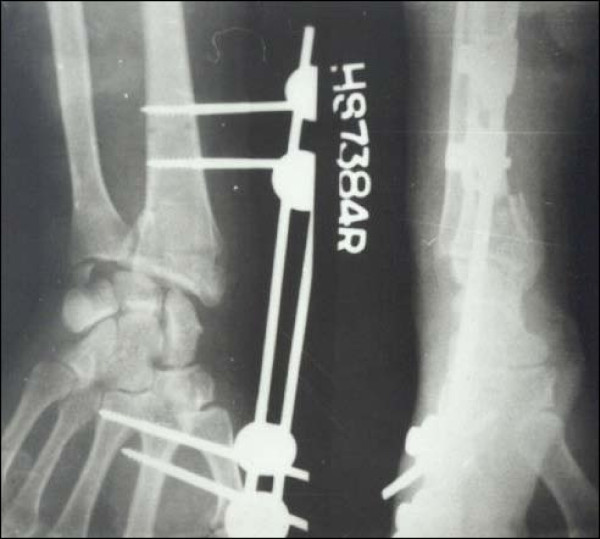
Case3 Postoperative Radiograph (Anteroposterior and Lateral view).
